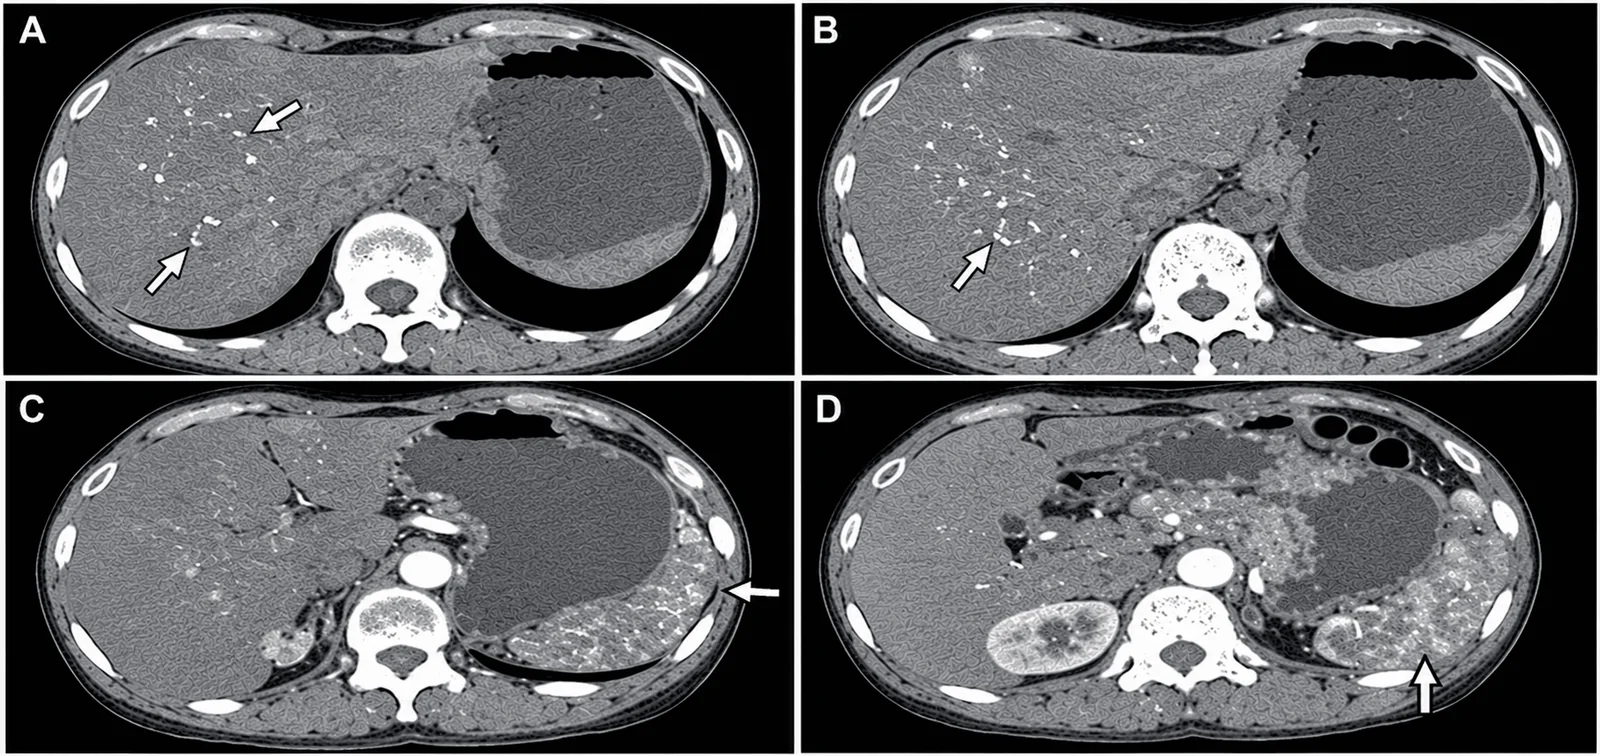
Contrast-enhanced CT scan of the abdomen and pelvis showing multiple calcified granulomas in the (A,B) liver (solid arrows) and (C,D) spleen (solid arrows)
